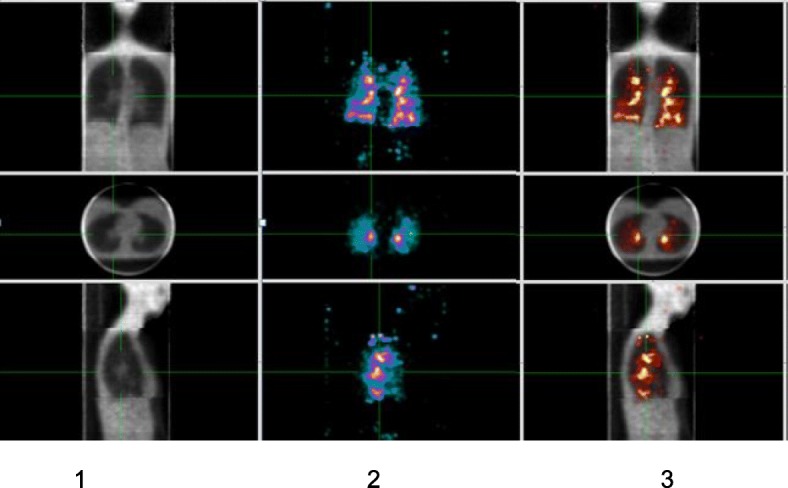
Representative images from one participant during simultaneous emission-transmission dynamic SPECT imaging of radioaerosol deposition: 1. The transmission data is primarily used for attenuation correction of the emission data 2. The emission data records the deposition of the inhaled radioaerosol 3. The transmission data is also used to segment the three-dimensional margin of the lung fields in order to determine the regional distribution of the deposited radioaerosol within the lungs
